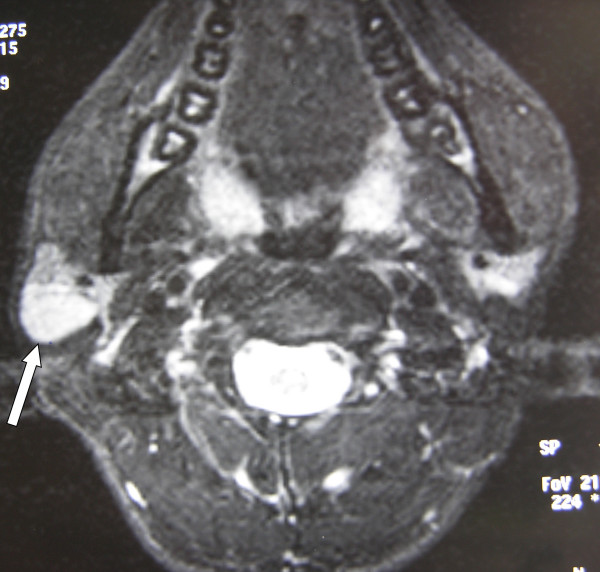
An axial fat saturated T2-weighted image through the parotid gland demonstrates a well-defined high signal intensity mass in the superficial area of the right parotid gland.
